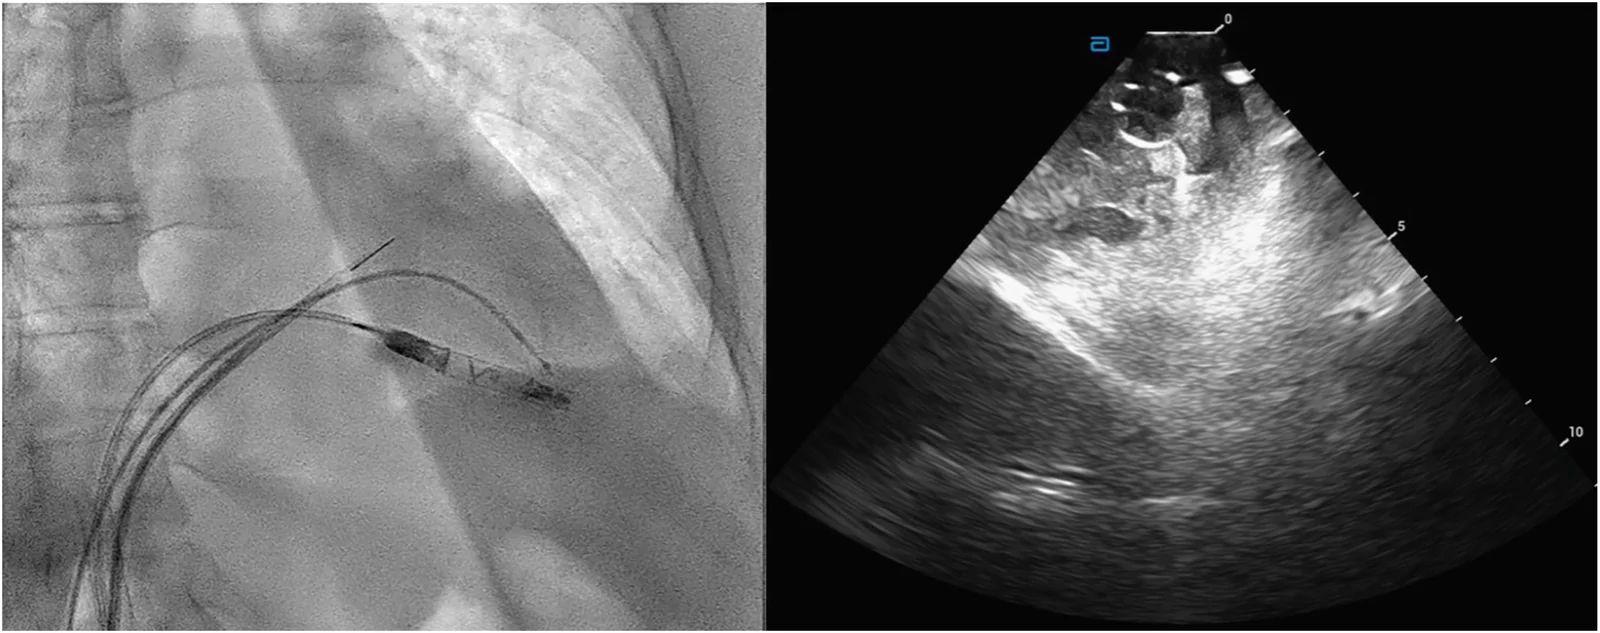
Fluoroscopic and intracardiac echocardiogram showing snaring of the leadless pacemaker.
